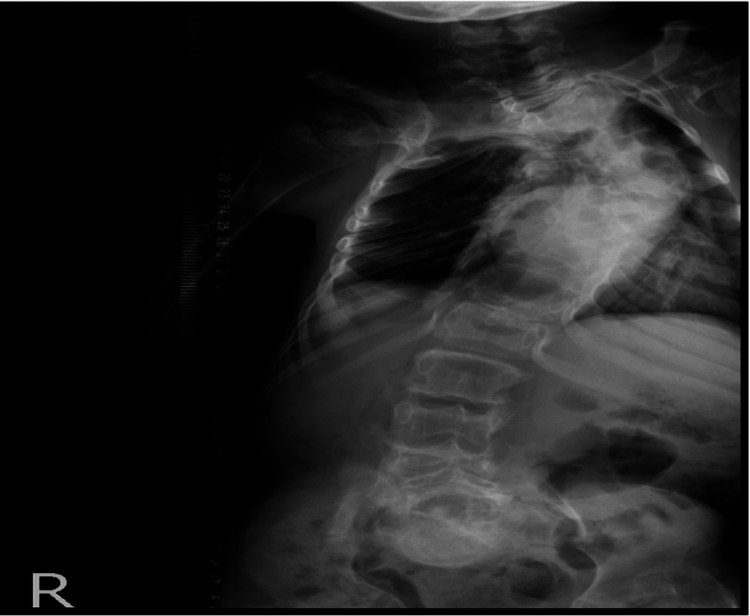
X-ray showed significant spinal kyphoscoliosis deformity
